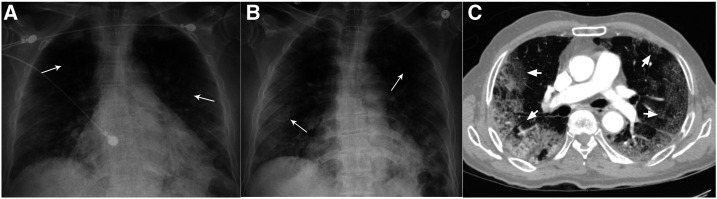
Chest radiography. (A) Chest X-ray on initial presentation showing bilateral peripheral patchy infiltrates (arrows). (B) Chest X-ray on readmission showing worsened bilateral peripheral patchy infiltrates (arrows). (C) Computed tomogram of the thorax showing peripheral-based extensive multi-lobar ground-glass opacities (arrows).
